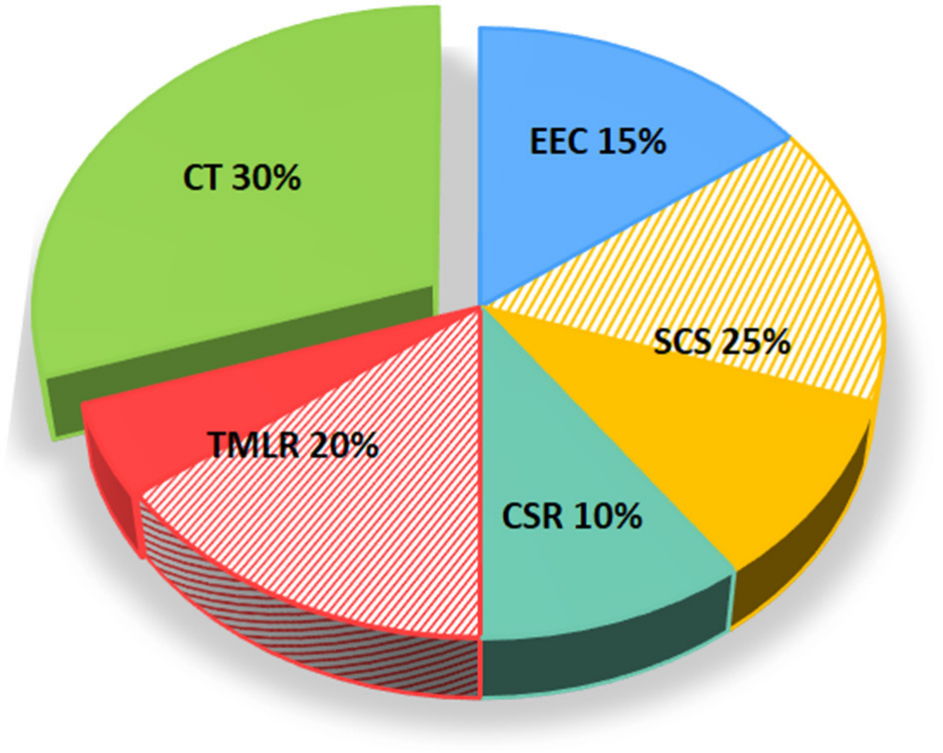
Meta-analyses and outcomes of non-pharmacological treatment options for refractory angina as per guidelines. The figure depicts the number and outcomes of available MTA for each non-pharmacological treatment options for RA including EEC, SCS, and CSR, in addition to CT. Positive MTA are represented with full color while those negatives are depicted with stripes. CSR, coronary sinus reducer; CT, cell-based therapy; EECP, enhanced external counterpulsation; MTA, meta-analysis; RA, refractory angina; SCS, spinal cord stimulation.
